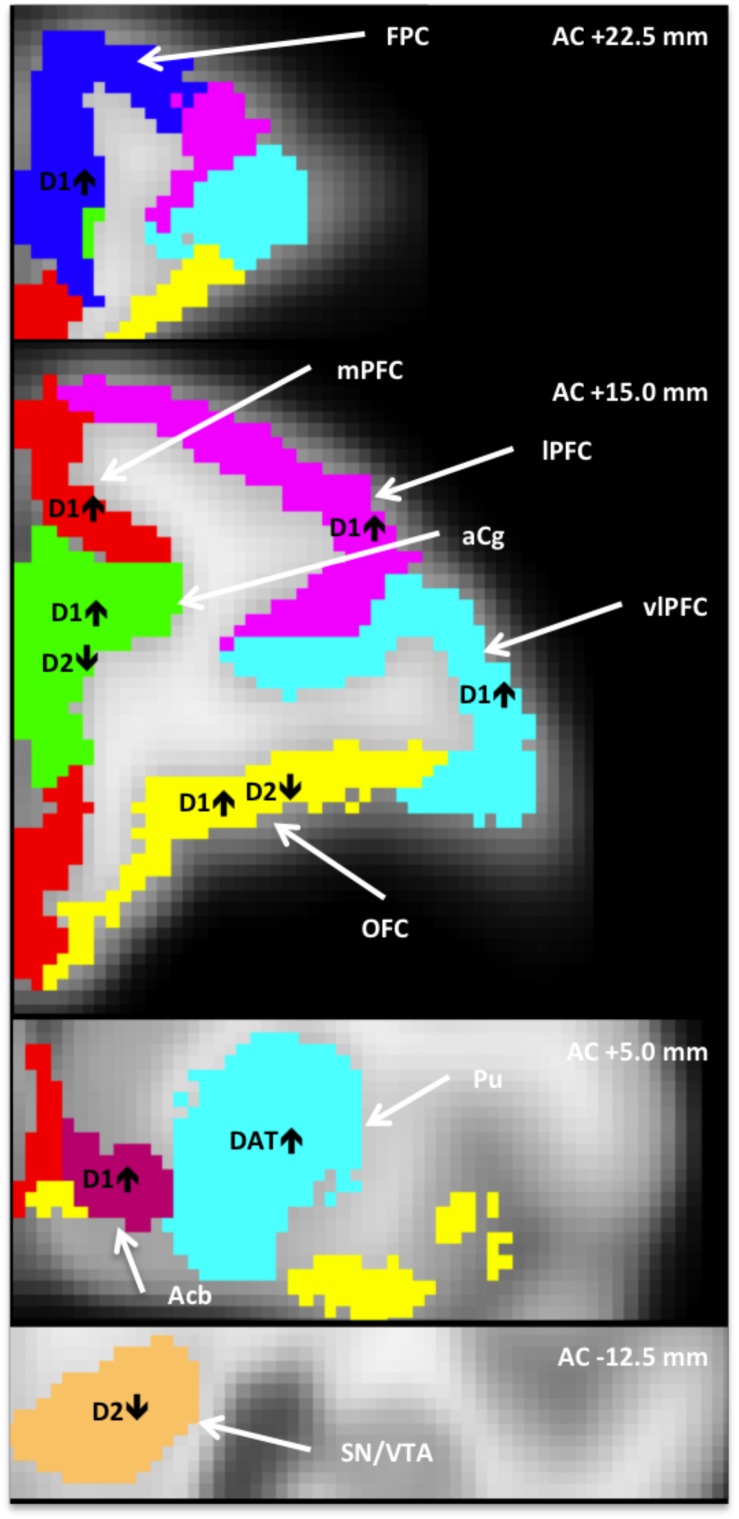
Anatomically defined regions overlaid on an MRI template (Moirano et al., 2018), in which dopaminergic measures correlated with heightened tactile responsivity (HTR). AC+/–, position relative to anterior commissure; Acb, nucleus accumbens; aCg, anterior cingulate; FPC, frontopolar cortex; lPFC, lateral PFC; mPFC, medial PFC; OFC, orbitofrontal cortex; vlPFC, ventrolateral PFC; PFC, prefrontal cortex; Pu, putamen; SN/VTA, substantia nigra/ventral tegmental area.
